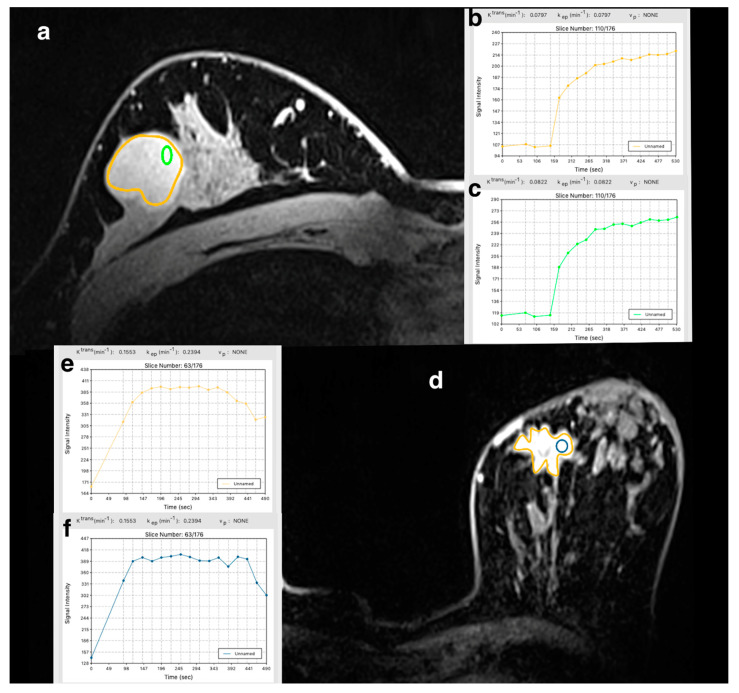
(a) A 31-year-old woman with fibroadenoma of the right breast on ultra-high-field dynamic contrast-enhanced magnetic resonance imaging at 7T. (b) Whole-tumor region of interest pharmacokinetic values and signal intensity curve. (c). Standard 10 mm^2^ ± 1 mm^2^ region of interest pharmacokinetic values and signal intensity curve. (d) A 49-year-old woman with invasive ductal carcinoma, luminal B, of the left breast on ultra-high-field dynamic contrast-enhanced magnetic resonance imaging at 7T. (e) Whole-tumor ROI pharmacokinetic values and signal intensity curve. (f) Standard 10 mm^2^ ± 1 mm^2^ region of interest pharmacokinetic values and signal intensity curve. Note: ROI: unnamed = no specific name was assigned to the ROI. Graph inserts are also provided separately as supplemental figures.
